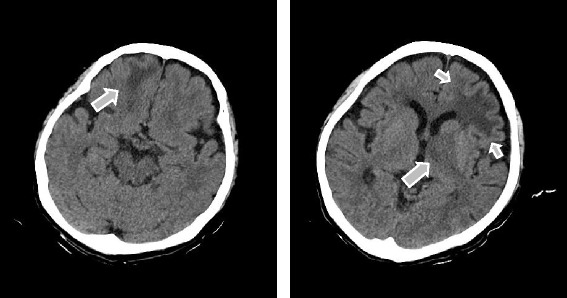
Cranial computed tomography scan on admission. Arrows indicated low density areas at the frontal lobes of both sides and the nucleus basalis of left side.
